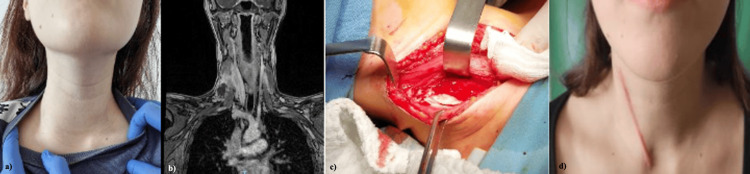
Deep cervical suppuration in a 17-year-old female patient after a viral episode of acute pharyngitis, with an altered immune terrain of autoimmune type. (a) Clinical picture. (b) MRI presurgical imaging. (c) Intraoperative appearance with right cervicotomy. (d) Final aspect at two weeks after discharge (our case).
